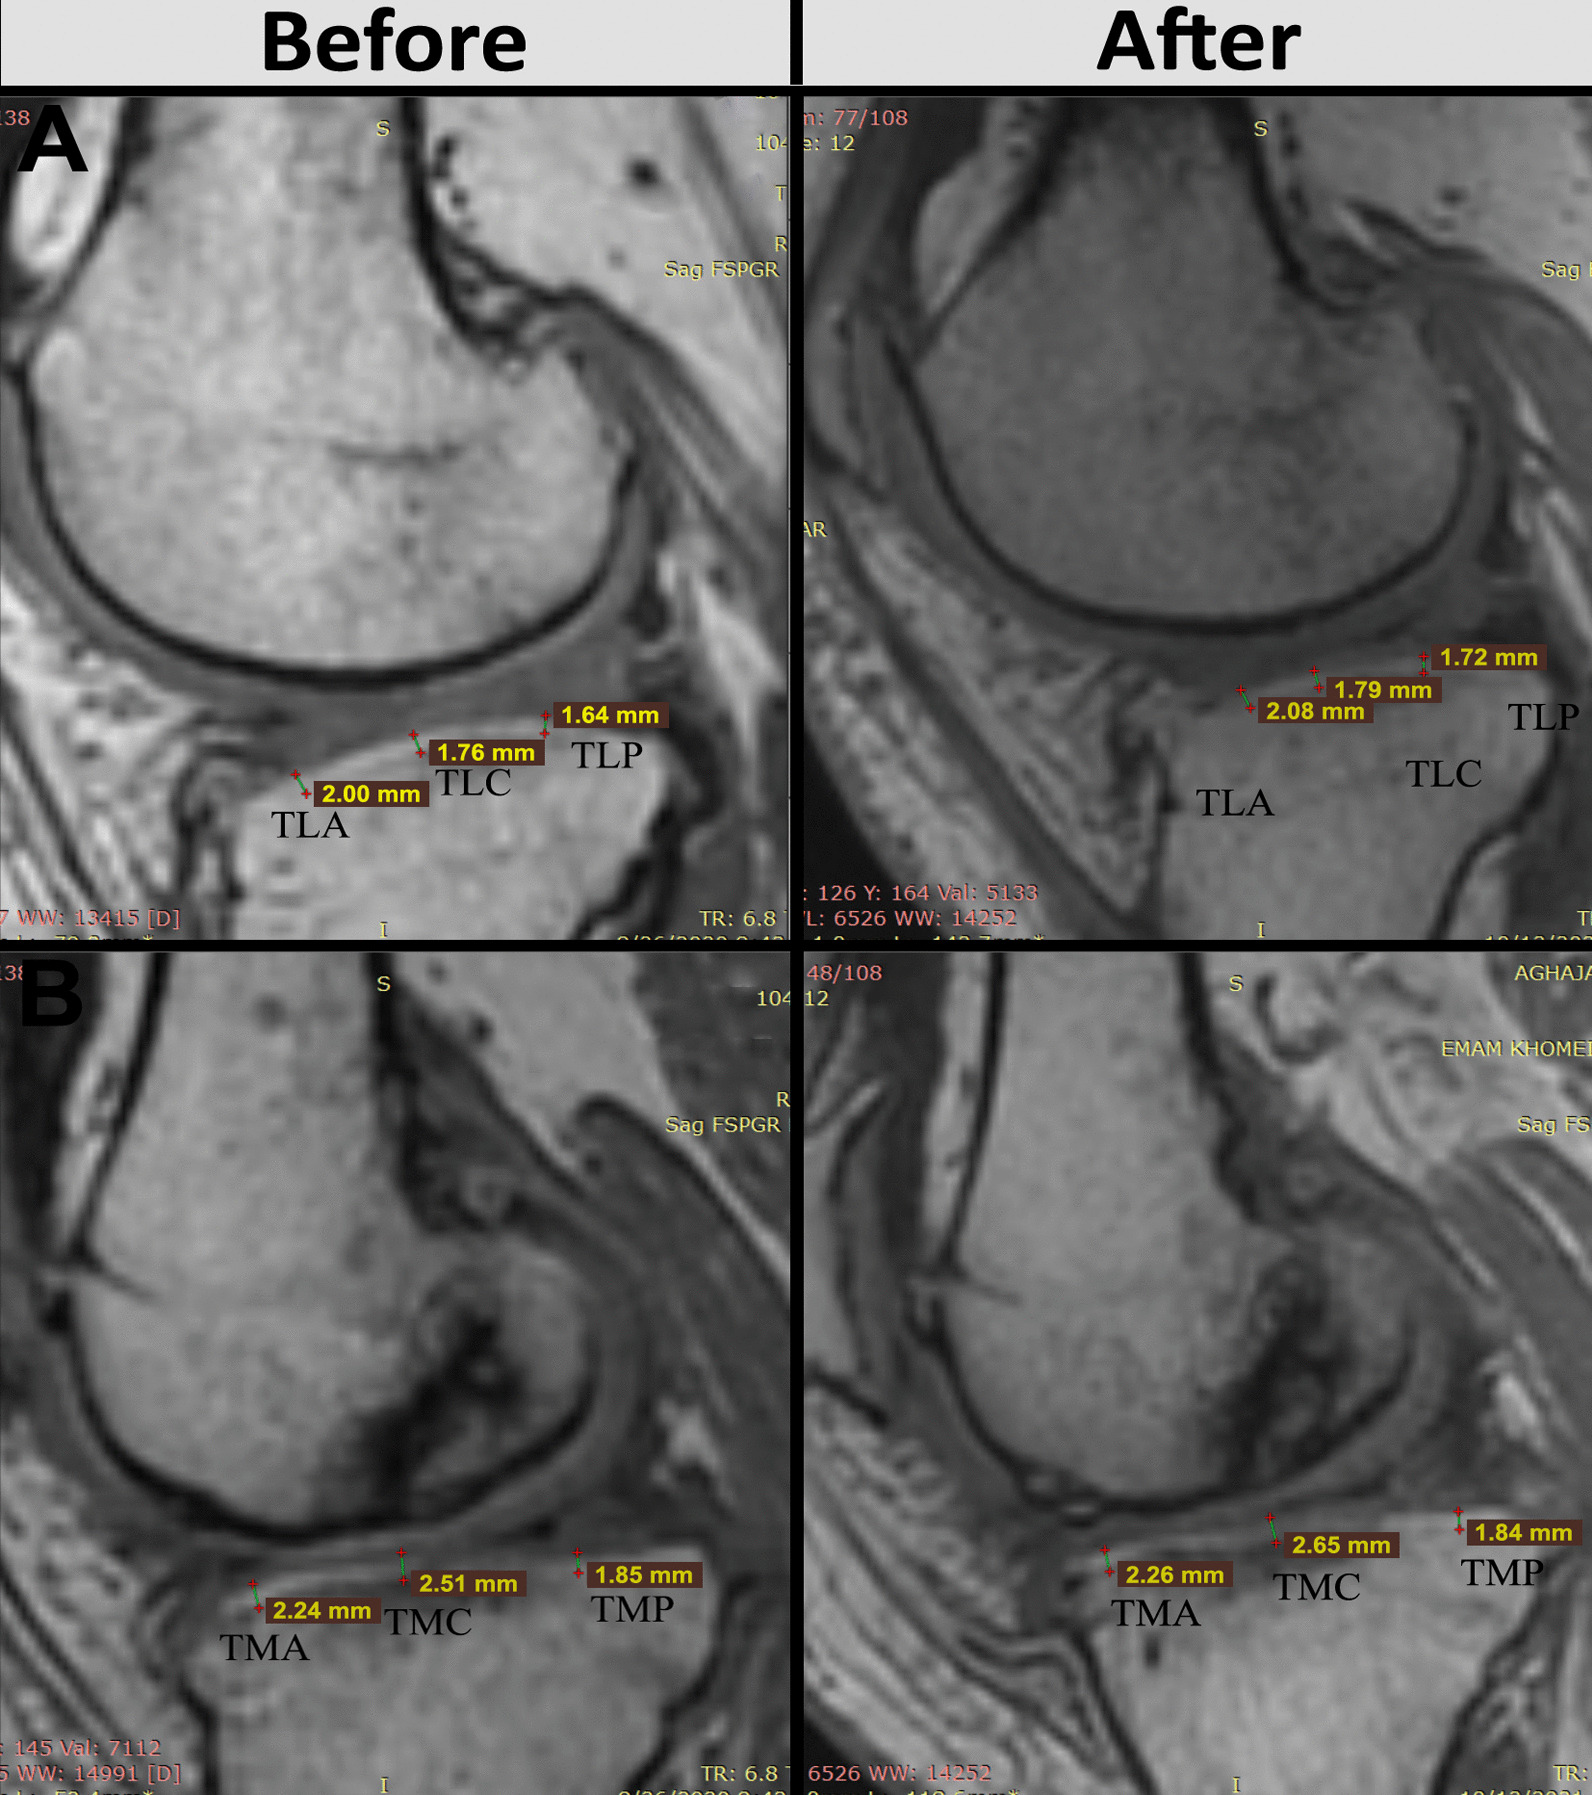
MRI analysis of tibial condyle cartilage before and 48 weeks after AD-MSCs injection. A Lateral radiograph indicates enhancement in anterior, posterior, and central area of tibial condyle cartilage. B Medial radiograph revealed increase in the thickness of tibial condyle cartilage in anterior, posterior, and central area. *MRI* magnetic resonance imaging, *TLA* tibia lateral anterior, *TLC* tibia lateral central, *TLP* tibia lateral posterior, *TMA* tibia medial anterior, *TMC* tibia medial central, *TMP* tibia medial posterior, *AD-MSCs* adipose-derived mesenchymal stromal cells
